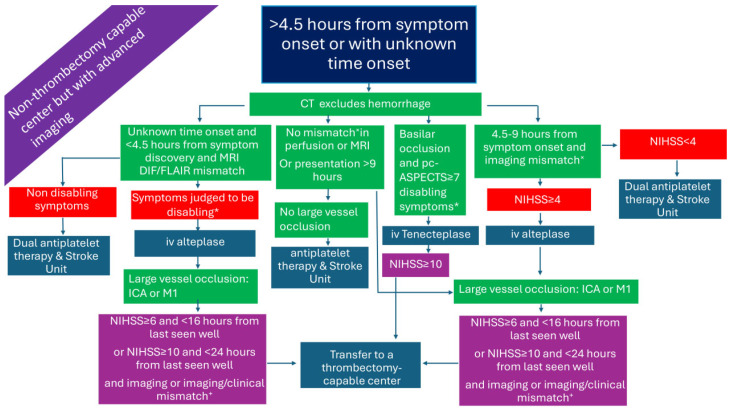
Management of AIS patients presenting >4.5 h from symptom onset in a non-thrombectomy-capable center but with advanced imaging. AIS: acute ischemic stroke; DIF/FLAIR: diffusion/Fluid-Attenuated Inversion Recovery; ICA: internal carotid artery; iv: intravenous; M1: M1 segment of the middle cerebral artery; M2: M2 segment of the middle cerebral artery; NIHSS: National Institutes of Health Stroke Scale; pc-ASPECTS: posterior circulation Alberta stroke program early CT score. * symptoms that interfere with daily living and previous activities of the patient. ^×^ Perfusion lesion–ischemic core mismatch ratio > 1.2, absolute difference in volume > 10 mL, and ischemic-core volume < 70 mL. ^+^ penumbral volume to infarct volume of ≥1.8, with an absolute penumbral volume of ≥15 mL and an estimated volume of the ischemic core < 70 mL (DEFUSE-3 [54]), or ≥80 years old and NIHSS ≥ 10 and infarct core < 21 mL, or <80 years old and NIHSS ≥ 10 and infarct core < 31 mL, or <80 years old and NIHSS ≥ 20 and infarct core 31 = 51 mL (DAWN [55]).
